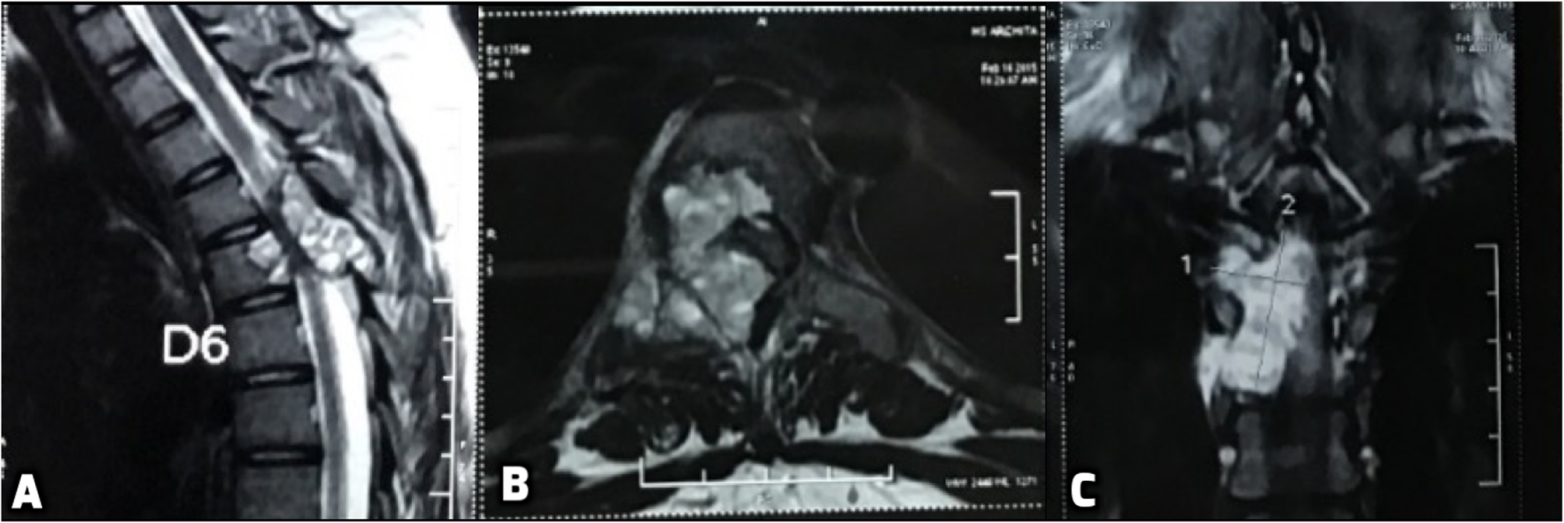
First Pre-operative MRI (A) revealing an intensely enhancing cystic cum haemorrhagic septate expansile bony lesion (5.7 × 3.1 cm) involving the posterior part of D5 vertebral body, right lamina, right pedicle and transverse process with epidural extension (D4–D5) causing marked spinal cord compression and underlying cord oedema. (B) Axial T2 MRI scan depicts the encroachment of the right half of body and posterior elements with compression of the spinal cord. (C) Coronal image through the spinal canal showing intense enhancement on T2 image with fluid levels.
